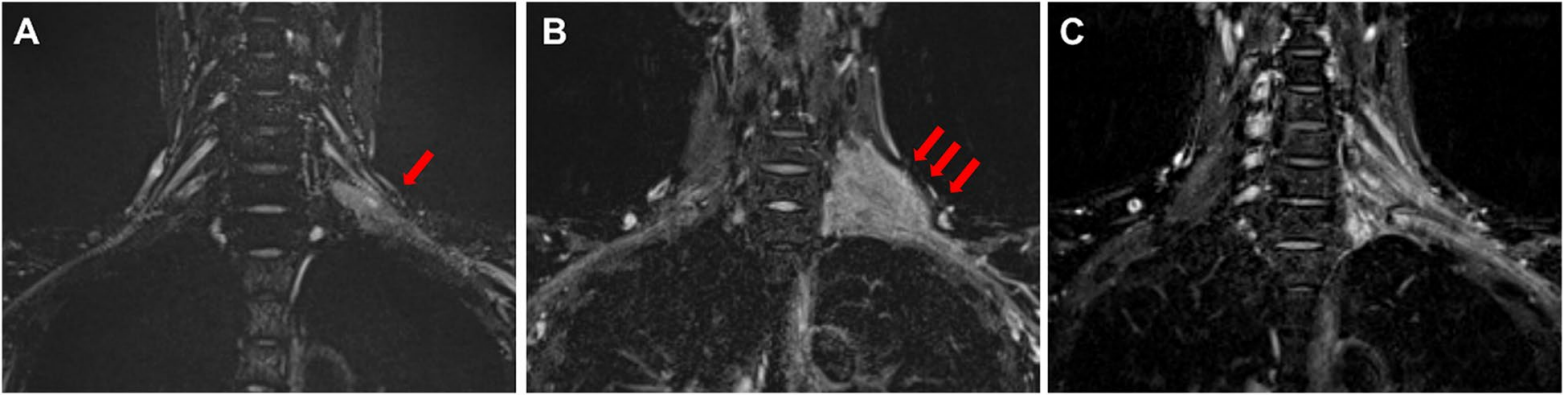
Magnetic resonance imaging of the brachial plexus. A Coronal short inversion time inversion recovery (STIR)-weighted magnetic resonance imaging (MRI) with contrast performed 38 days after the onsent of pain in her extremities shows hyperintense signals of the brachial plexus swelling (arrow) with enhancement at diagnosis. B Coronal STIR-weighted MRI performed 3 months after pain onset shows enlarged mass lesion. C Coronal STIR-weighted MRI performed 40 days after the completion of radiation therapy shows significant tumor shrinkage. MRI: magnetic resonance imaging, STIR: short inversion time inversion recovery
